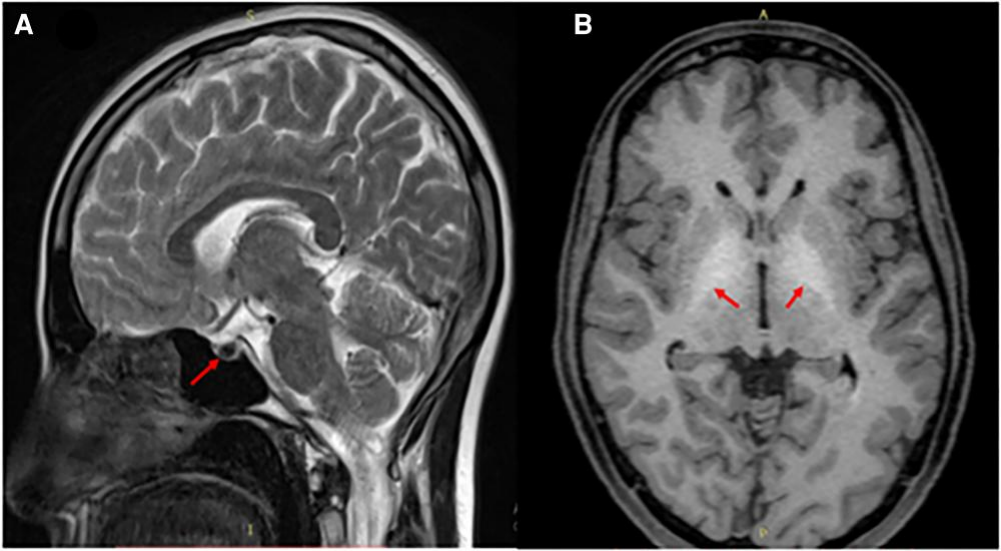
(A). T2-weighted MRI showing hypointensity of the pituitary gland, consistent with iron deposition. (B). T1-weighted MRI showing bilateral globus pallidus hyperintensity, also resulting from iron overload.
